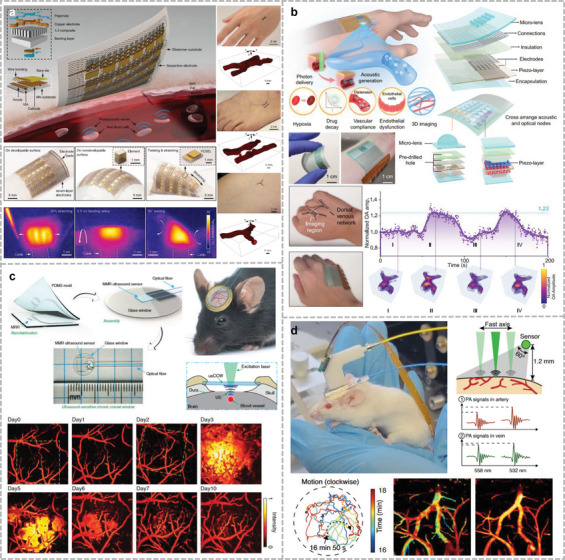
Wearable photoacoustic imaging. a) A flexible patch integrating an ultrasound piezoelectric unit and a vertical cavity surface emitting laser (VCSEL) allows the reconstruction of a three‐dimensional map of hemoglobin with sub‐millimeter resolution. Reproduced under the terms of CC‐BY license.^[^
